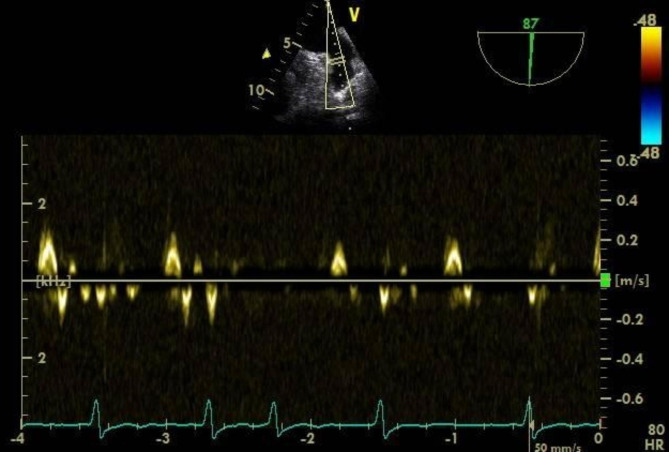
Measurement of left atrial appendage emptying velocity by pulse Doppler. The emptying velocity of left atrial appendage was 20 cm/s
